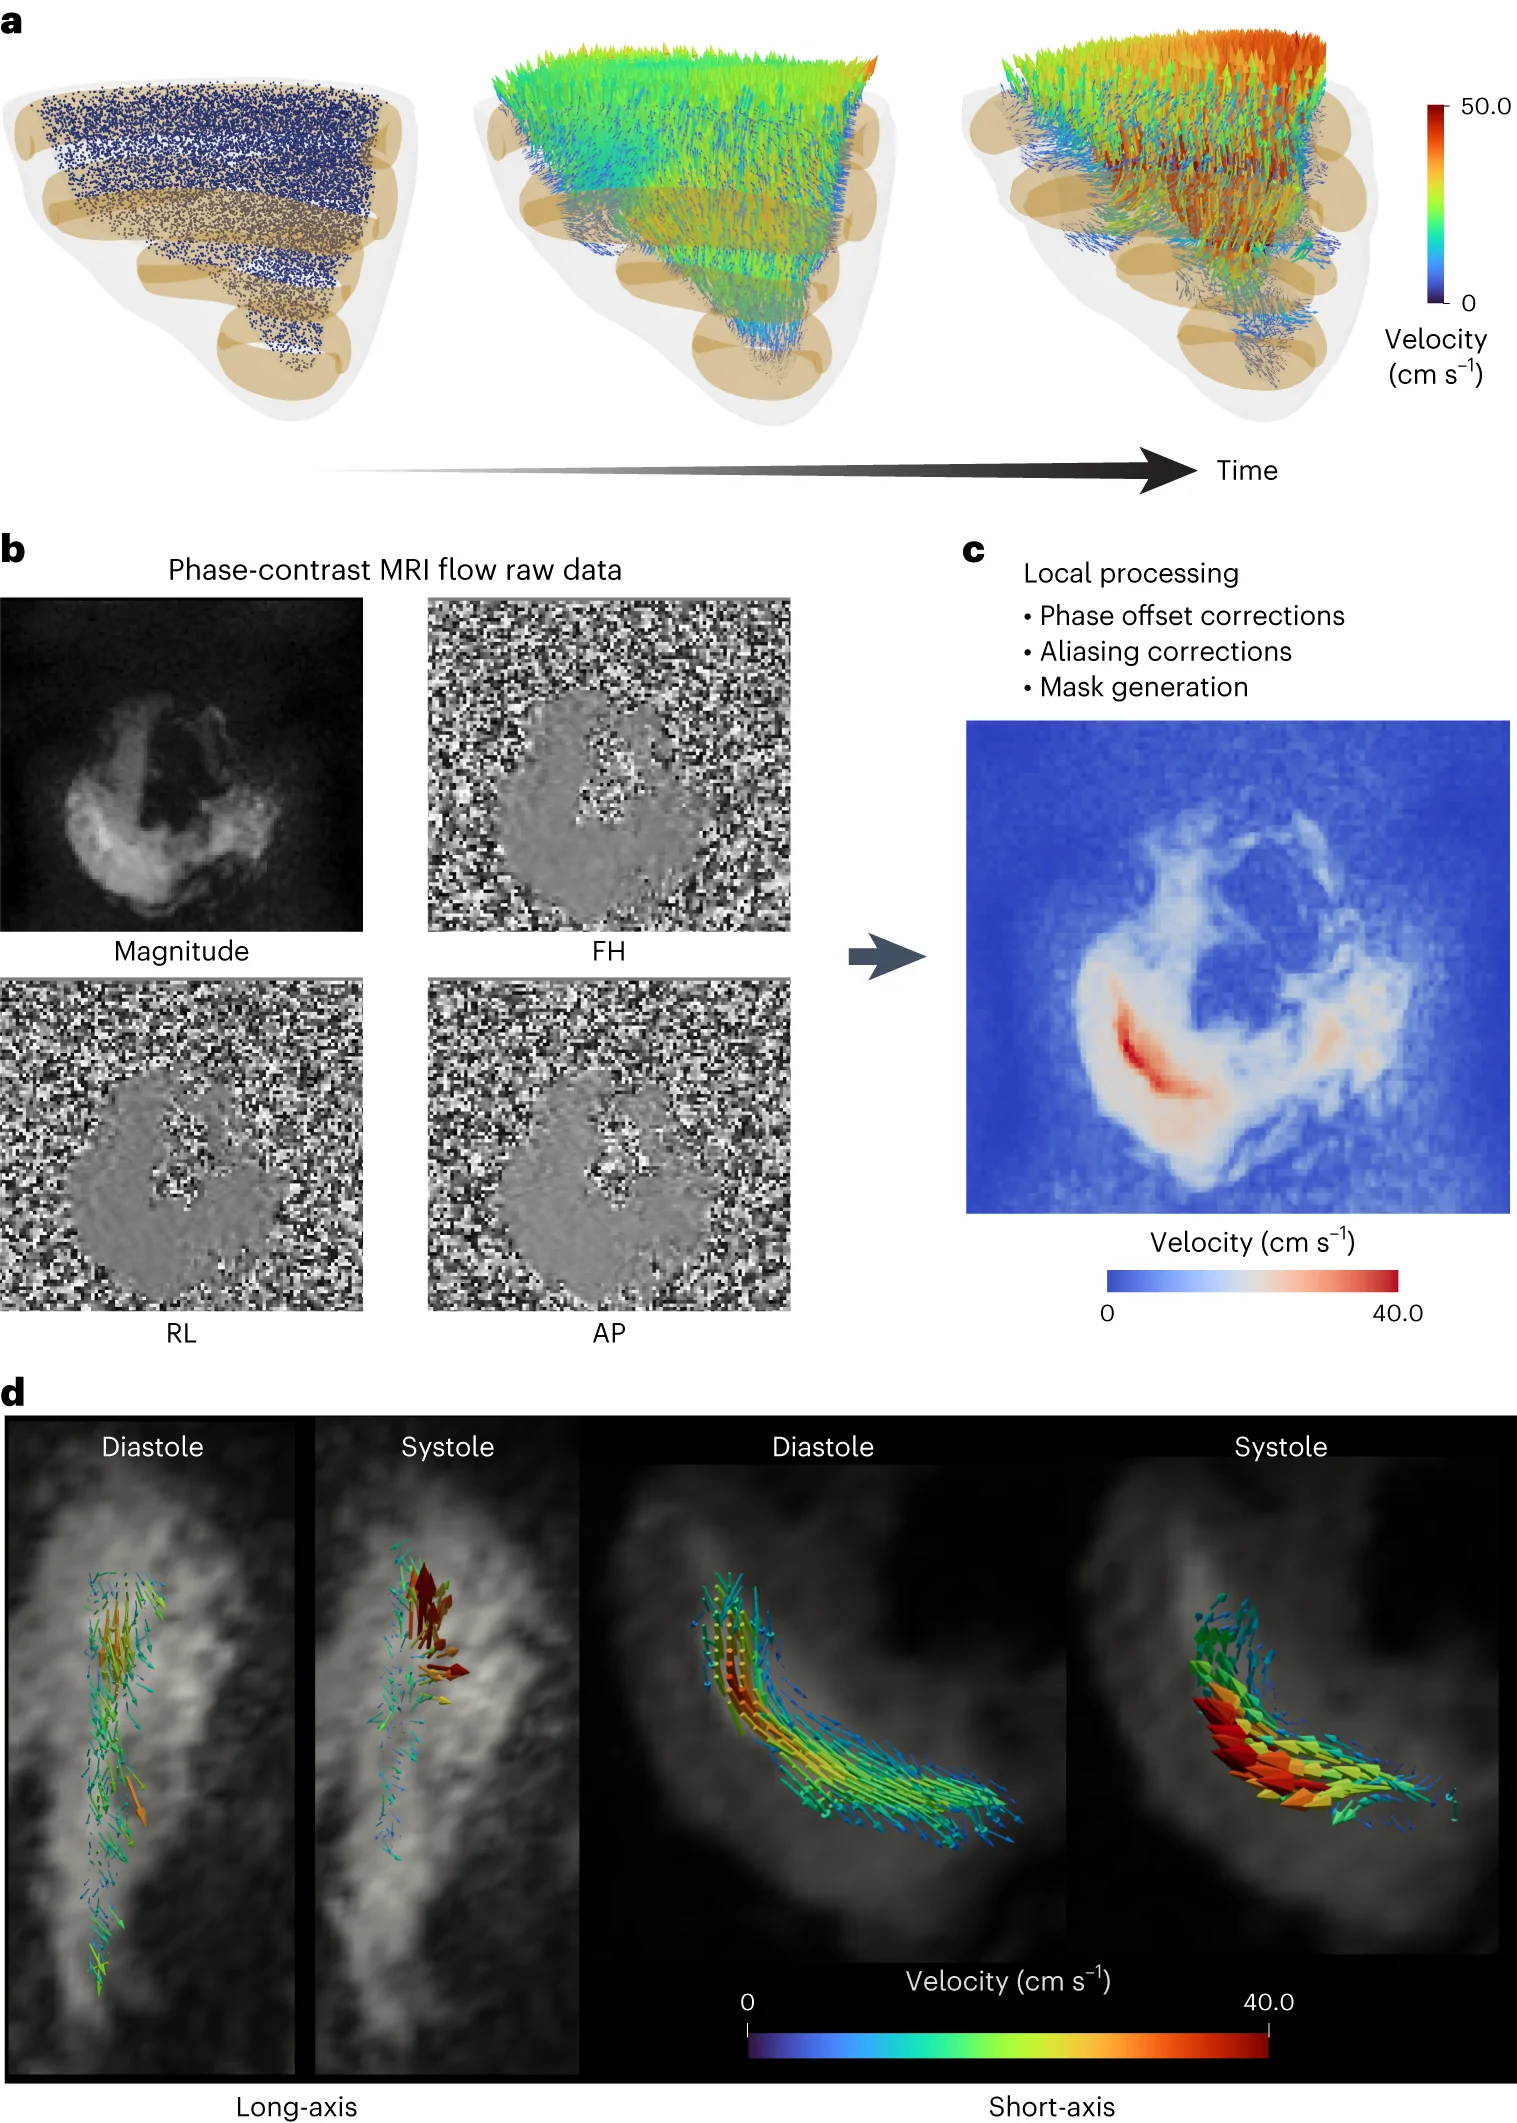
CFD models and MRI velocimetry. a, Computational fluid model to predict the interventricular fluid particle velocity. b, The magnitude and phase data of a 2D MRI flow measurement sequence are depicted. AP, anterior-posterior direction; FH, foot–head; RL, right–left. c, The cross-sectional data recorded during the study were carefully analyzed and corrected to accurately depict the distribution of flow across the RV. d, Reconstructed velocity vectors superimposed on the long-axis and short-axis cine images during both systole and diastole.
